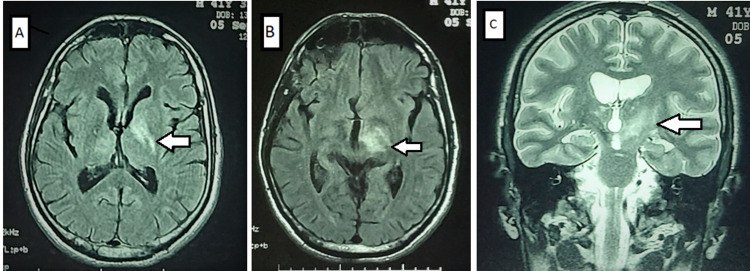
Brain MRI. Axial fluid-attenuated inversion recovery (FLAIR) sequences demonstrated the increased signal intensity of the left internal capsule (A) with the lesion of the left anteromedial thalamus (B). Coronal T2-weighted sequence (C) demonstrated the high-signal intensity of the left meso-diencephalic region. Location of cerebral lesions (white arrow).
